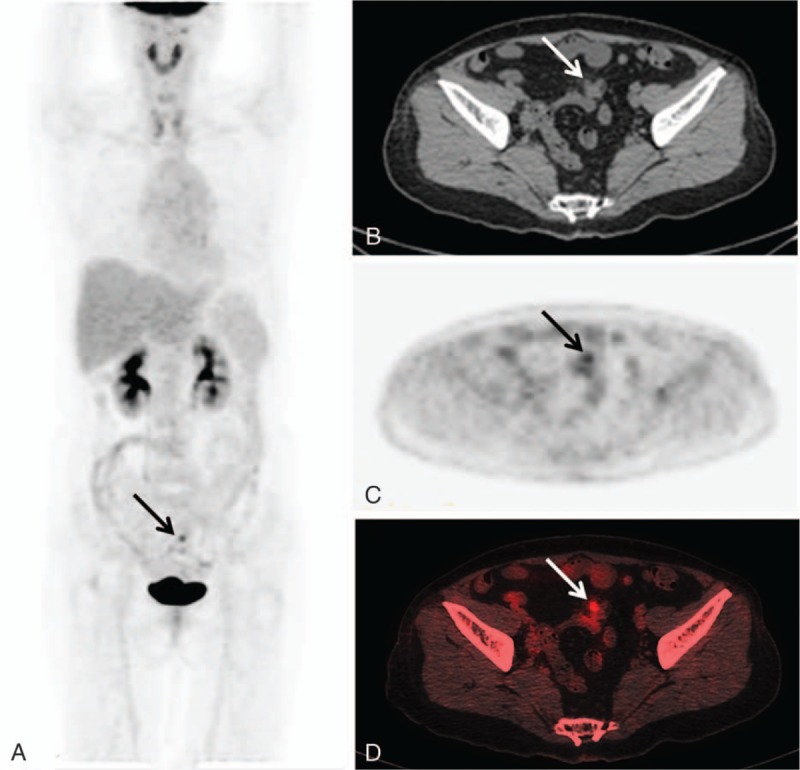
The maximum intensity projection PET image of ^18^F-FDG PET/CT scan (A) revealed a focal increased ^18^F-FDG uptake lesion (arrow) and normal ^18^F-FDG uptake of the pancreas. Transverse CT (B), and corresponding PET (C) and fusion (D) images showed the lesion (arrow) with the SUV_max_ of 4.3 in the small intestine. CT = computed tomography,^18^F-FDG PET = ^18^F-fluorodeoxyglucose positron-emission tomography, SUV_max_ = maximum standardized uptake value.
